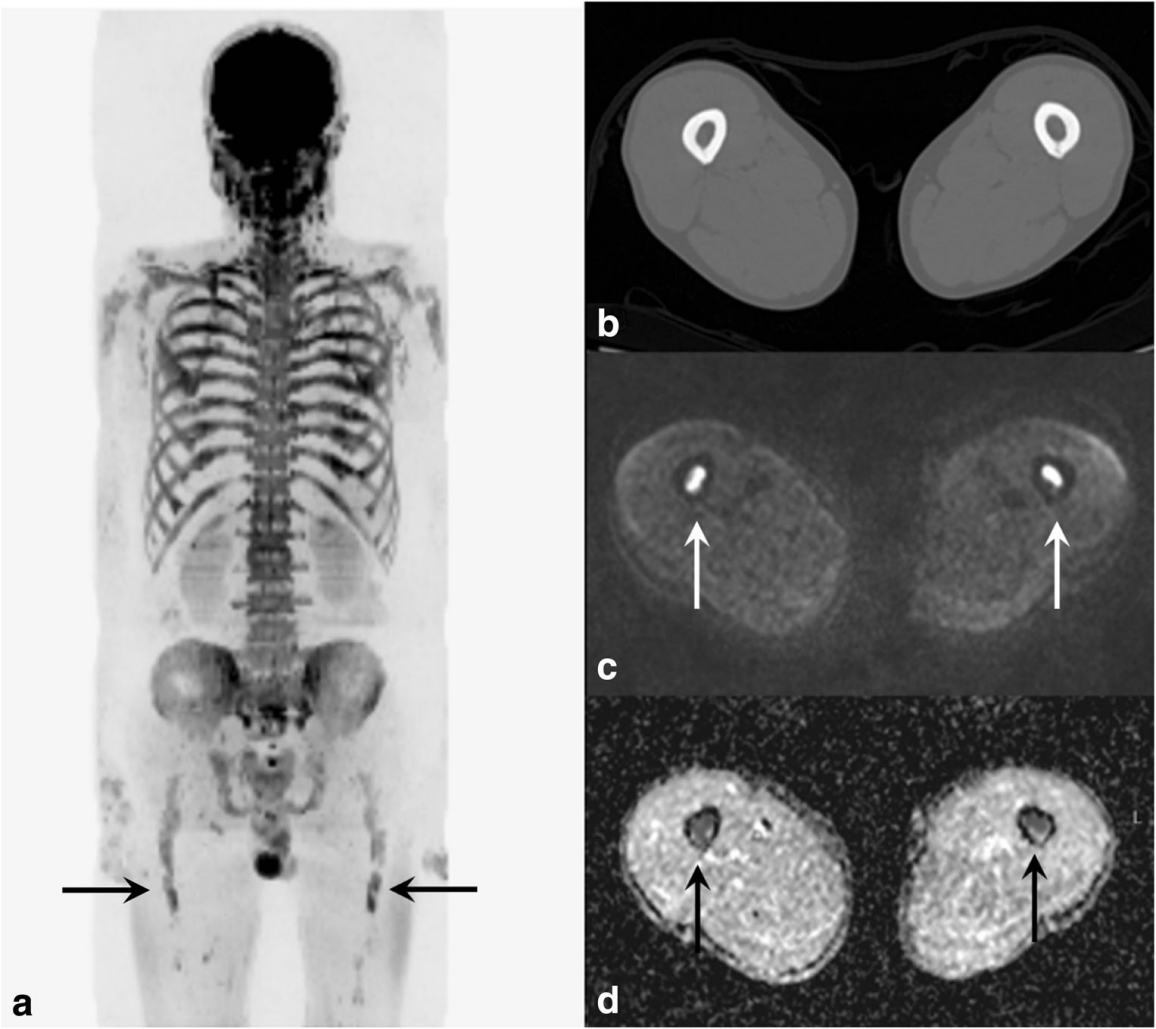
Marrow infiltration in bilateral femora in a 67-year-old male patient with IgG kappa myeloma and a high disease burden of 70% clonal cells on bone marrow biopsy. Disease is occult on axial CT (b) as there is no cortical destruction. However, widespread marrow disease is easily appreciable on WB-MRI maximum intensity projection (a, black arrows), *b* = 900 s/mm^2^ (c, arrows) axial images and ADC map (d, arrows), as regions of low signal intensities on the WB-DWI MIP image and as foci of high signal on the native b900 DWI image
